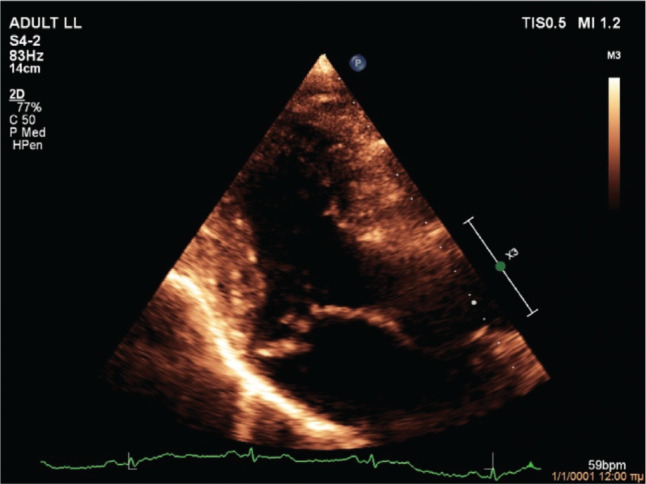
Case 2 echocardiogram showing bileaflet mitral valve prolapse (Barlow’s disease) with flail posterior leaflet.
